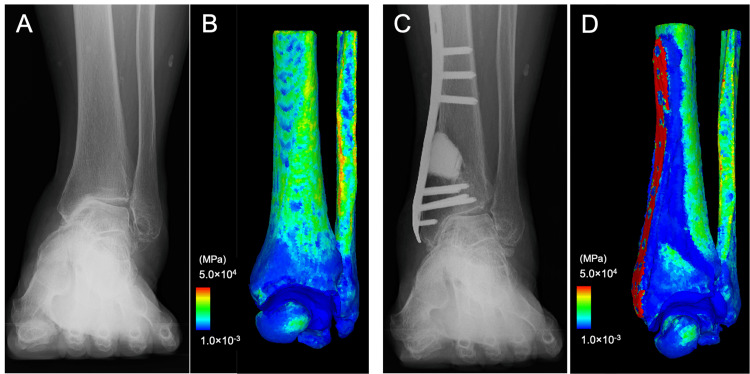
Case 1: A 73-year-old woman with stage 3a osteoarthritis of the left ankle. (A) preoperative anteroposterior weight-bearing radiograph of the ankle (B) the corresponding finite-element model with the distribution of the elastic modulus (C) postoperative anteroposterior weight-bearing radiograph of the ankle, and (D) the corresponding finite-element model with the distribution of the elastic modulus at six months after distal tibial oblique osteotomy
